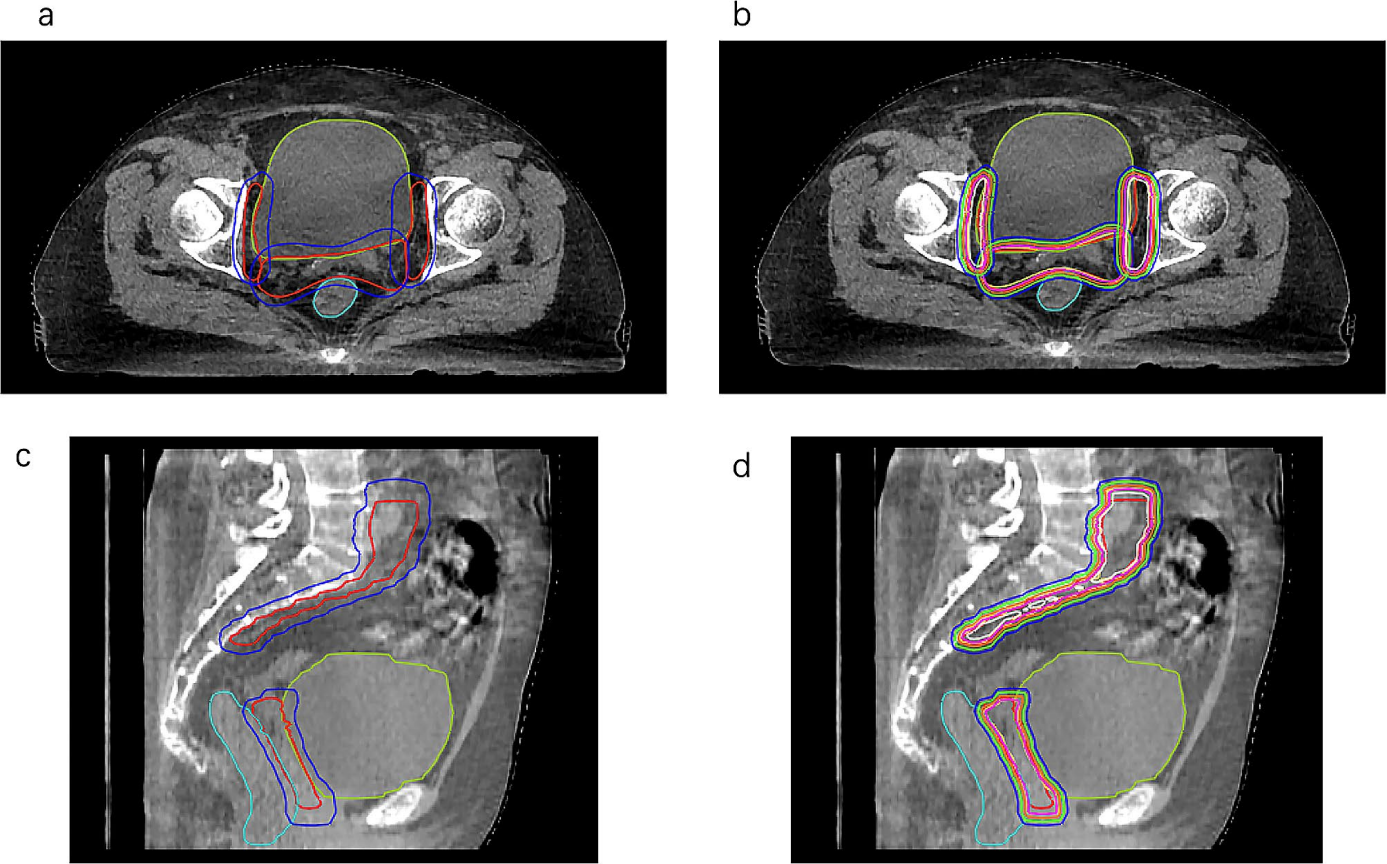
Example images of axial (a and b) and sagittal (c and d) slices of a patient from the validation cohort. The CTVpost (red) and PTVpre (blue) contours were superimposed onto the images after pre- and posttreatment iCBCT matching with respect to bony anatomy. After 100% coverage with a 5 mm margin (a and c), margins of 0 (CTVpre, yellow), 1 (white), 2 (magenta), 3 (orange), 4 (green), and 5 (PTVpre, blue) mm in the three-dimensional direction were explored (b and d)
